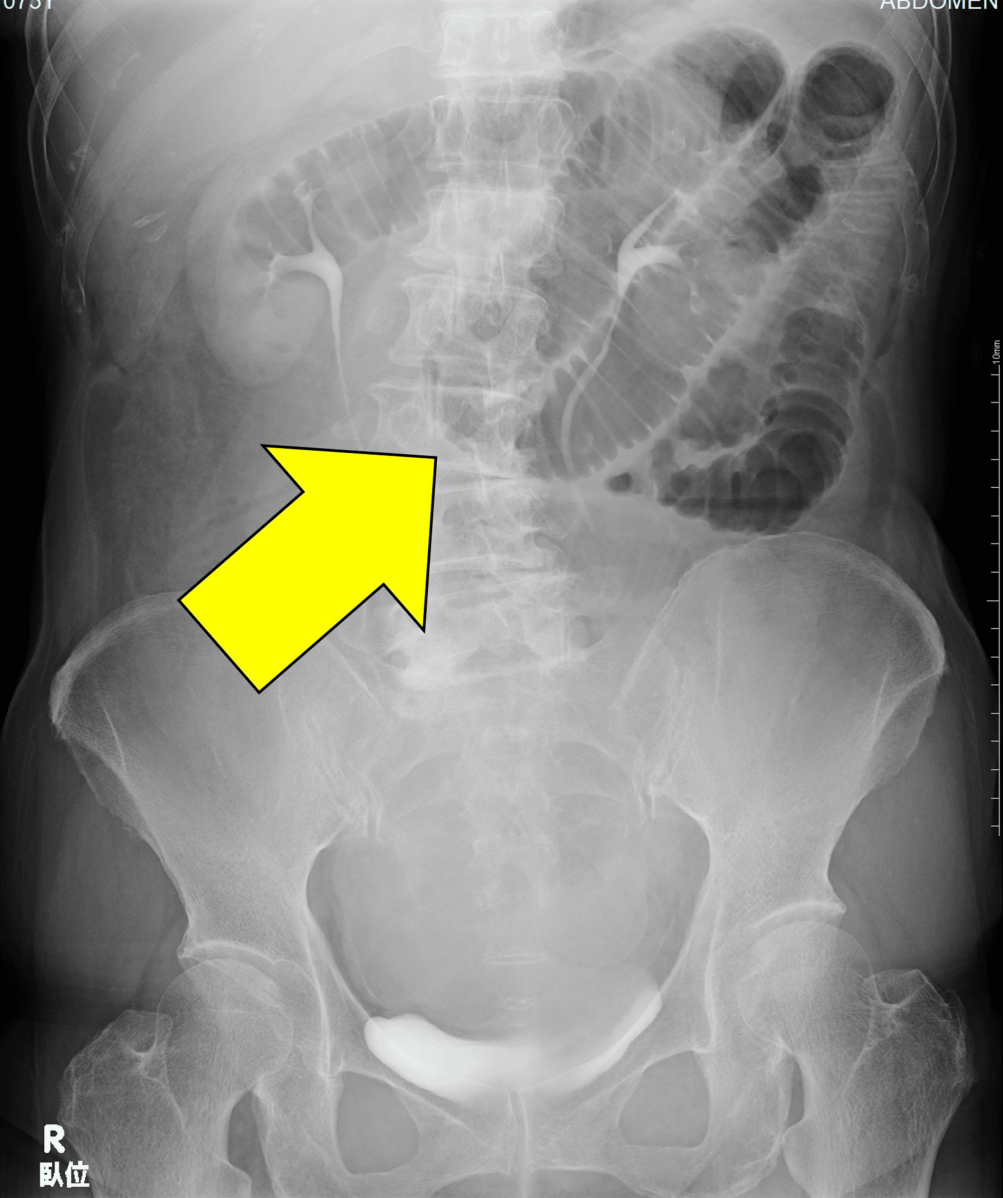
Abdominal plain X-ray revealed small bowel dilation (supine position).
